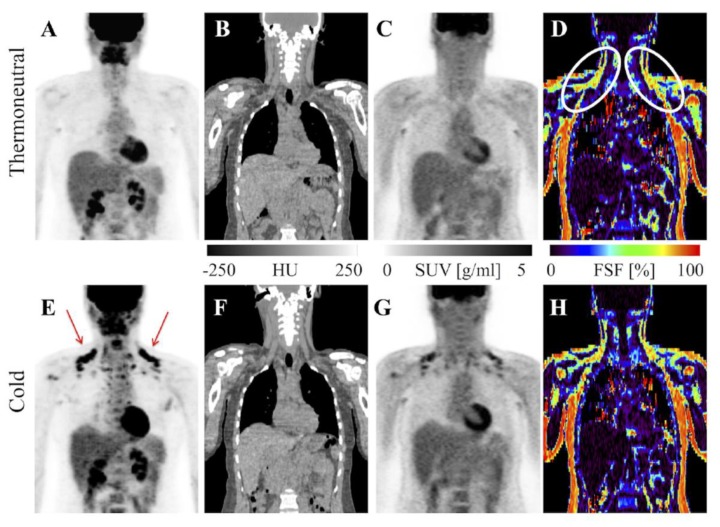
MRI vs. FDG-PET/CT in detection of BAT. FDG-PET/CT and MRI of a healthy BAT-positive research subject. FDG uptake is visible in supraclavicular BAT with cold exposure on the PET maximum intensity projection (MIP; E, red arrows) and absent from the thermoneutral PET MIP (A). CT shows adipose tissue in the same region (B,F) and PET shows increased standardized uptake value there after exposure to cold (C,G). MRI-derived fat signal fraction from the same subject shows adipose tissue with relatively low levels of fat (white ovals) in the same region at thermoneutral and cold conditions (D,H). Image reproduced with permission from reference 41.
